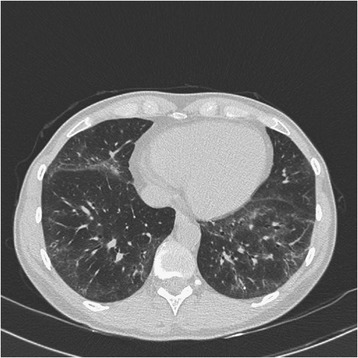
High-resolution computed tomography image. Image compatible with nonspecific interstitial pneumonia showing ground-glass opacities in both lung bases.
